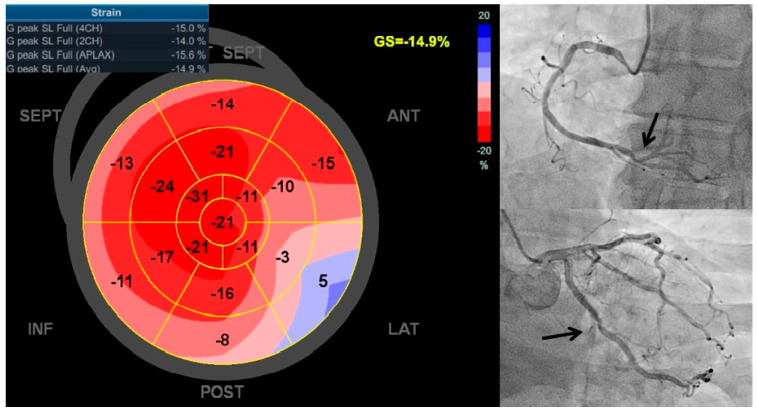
A 64-year-old male presented with exertional chest pain and was referred for evaluation of suspected coronary artery disease. Resting echocardiography showed preserved left ventricular ejection fraction (LVEF), but a markedly reduced GLS of 14.9% (image on the left). Coronary angiography revealed a 70% stenosis in the right coronary artery (image on the right top) and complete (100%) occlusion of the left circumflex artery (image on the right bottom).
